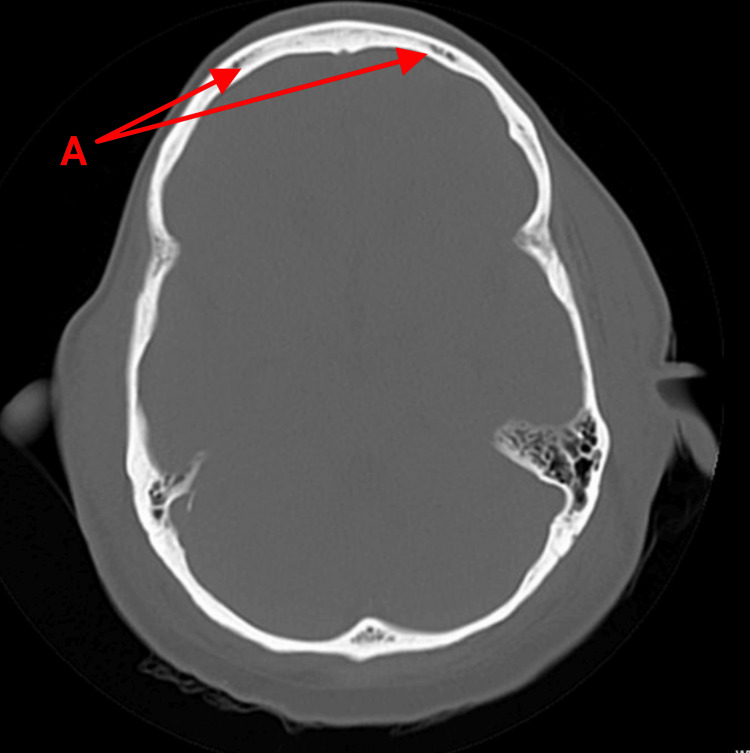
Computed tomography (CT) scan of the head A: The arrows originating from the letter "A" (highlighted in red) indicate patchy areas of the calvarium that are consistent with metastatic disease.
